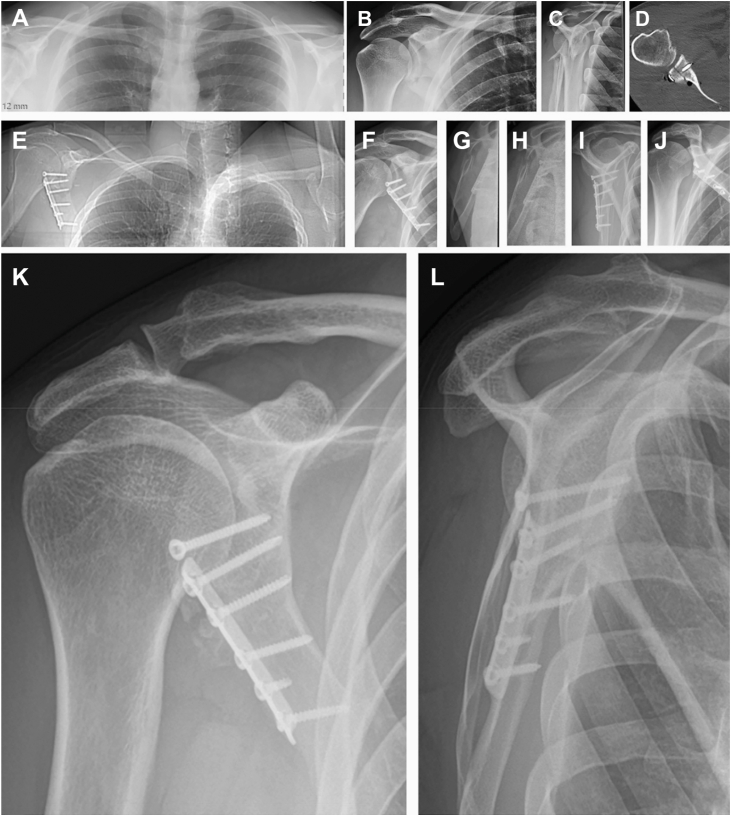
Single disruption injury of SSSC and its treatment. A right scapular fracture involving the glenoid cavity and scapular neck occurred in a 42-year-old patient following a motorcycle accident. (A) Panoramic radiograph of the clavicle. (B) Anteroposterior radiograph of the right shoulder. (C) Scapular Y-view radiograph of the right shoulder. (D) Axial CT image at the level of the glenohumeral joint demonstrating a scapular shaft fracture extending into the superior portion of the glenoid cavity. (D–H) Immediate post-operative radiographs (axial CT in D) following open reduction and internal fixation using a 5-hole one-third tubular plate and a free 3.5 mm cortical screw. (I and J) Radiographs at 4-month follow-up, showing stable fixation and ongoing fracture healing. (K and L) Radiographs at 4-year follow-up, demonstrating full fracture union and maintained alignment without implant-related complications. Although single disruptions are usually managed conservatively, the presence of glenoid involvement and the potential for articular or mechanical compromise justified operative fixation in this case. Open reduction and internal fixation using a 5-hole one-third tubular plate and a free 3.5-mm cortical screw achieved complete fracture union and maintained alignment at 4-year follow-up without implant-related complications. Therefore, the key treatment question in single disruptions is not simply whether one structure is injured, but whether that injury preserves or compromises the functional stability of the shoulder girdle. *SSSC*, superior shoulder suspensory complex; *CT*, computed tomography.
